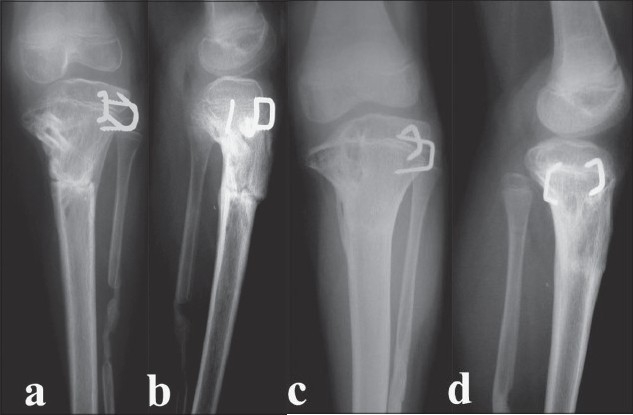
Anteroposterior and lateral (a,b) radiographs taken two months postoperatively. The osteotomies have united, the medial tibial plateau has been satisfactorily elevated and the cortical strut grafts are consolidating well. Anteroposterior and lateral (c,d) radiographs taken two years postoperatively showing maintenance of correction and excellent alignment. There is no recovery of growth in the medial tibial physis
